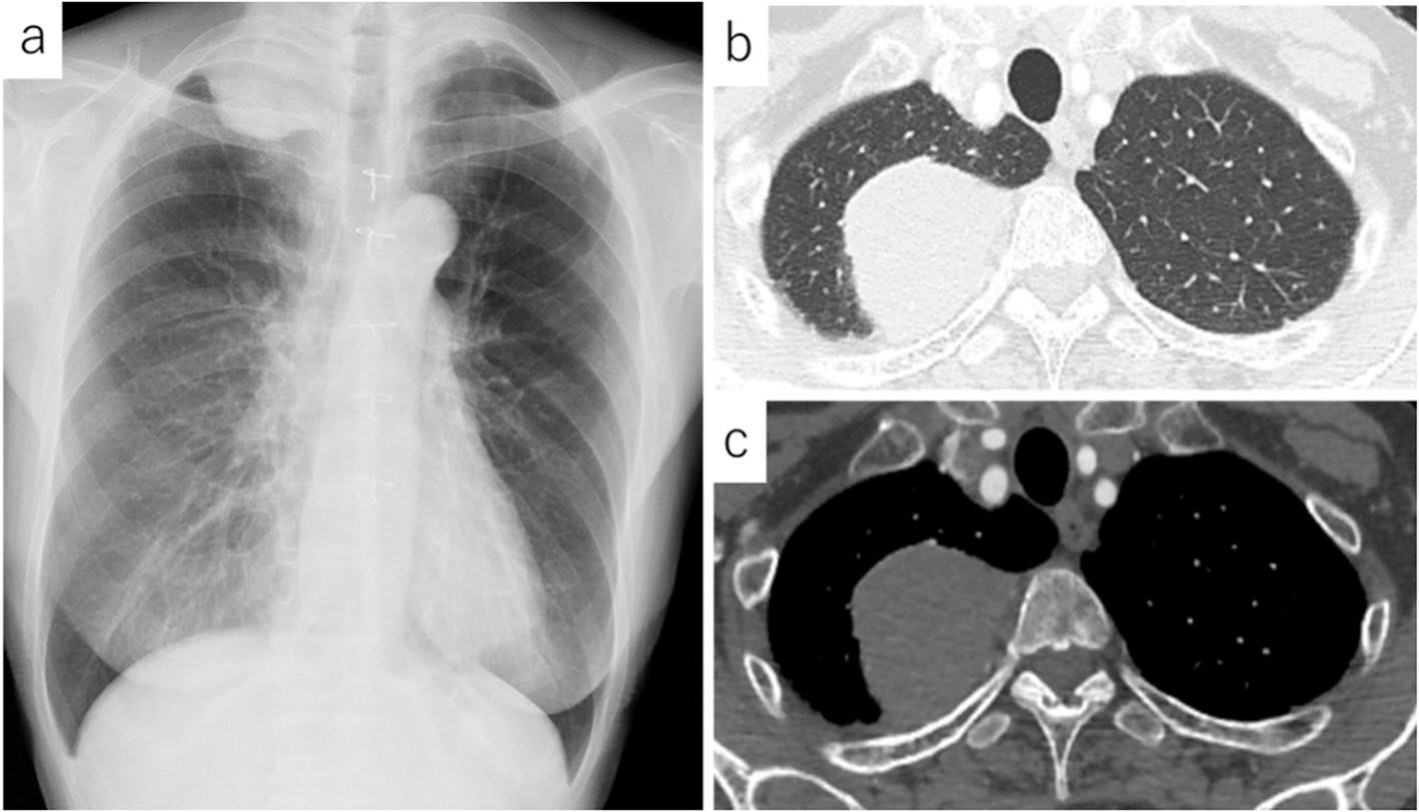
a Chest X-ray image 21 years after complete removal. A solid mass with a clearly defined border at the right apex is observed. b Enhanced chest computer tomography scan 21 years after complete removal. A 60-mm solid mass with a clearly defined rim in the right upper lobe is observed
